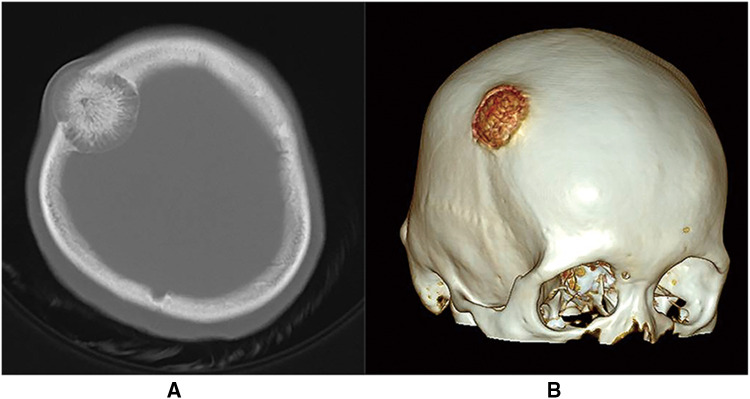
(A) Cranial CT scan (bone window) showing a right frontoparietal junctional area mass with distending growth, accompanied by osteolytic changes, showing the phenomenon of “daylight radiation”. (B) Cranial CT 3D reconstruction.
